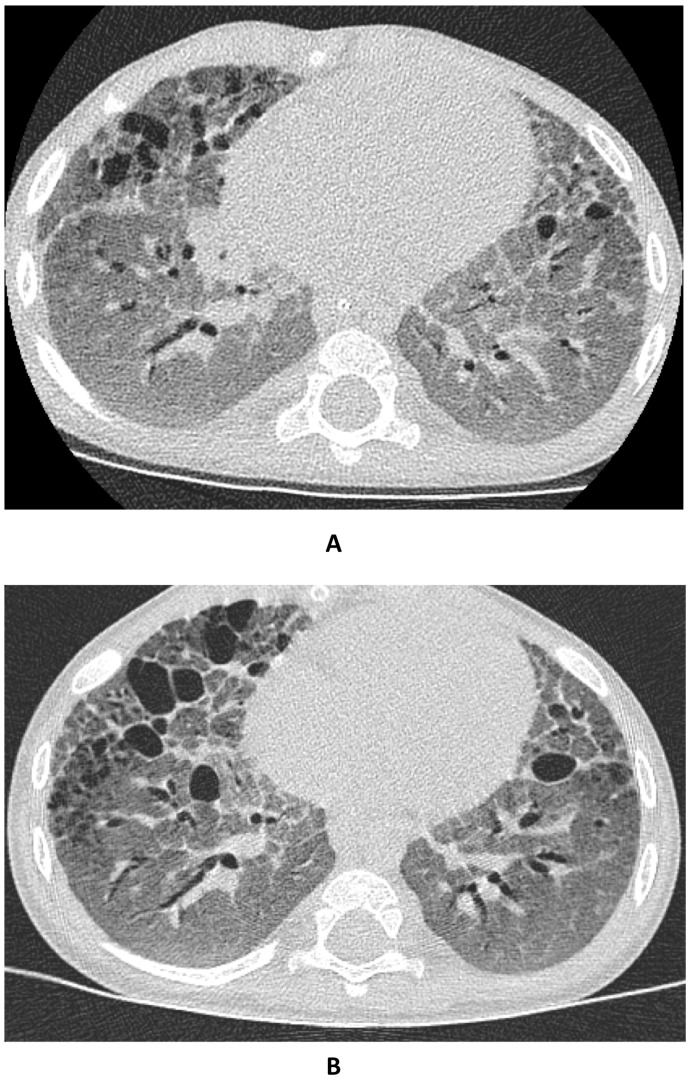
High-resolution CT imaging of pulmonary aspect before (A) and after (B) infusion 3 of mesenchymal stromal cells.
